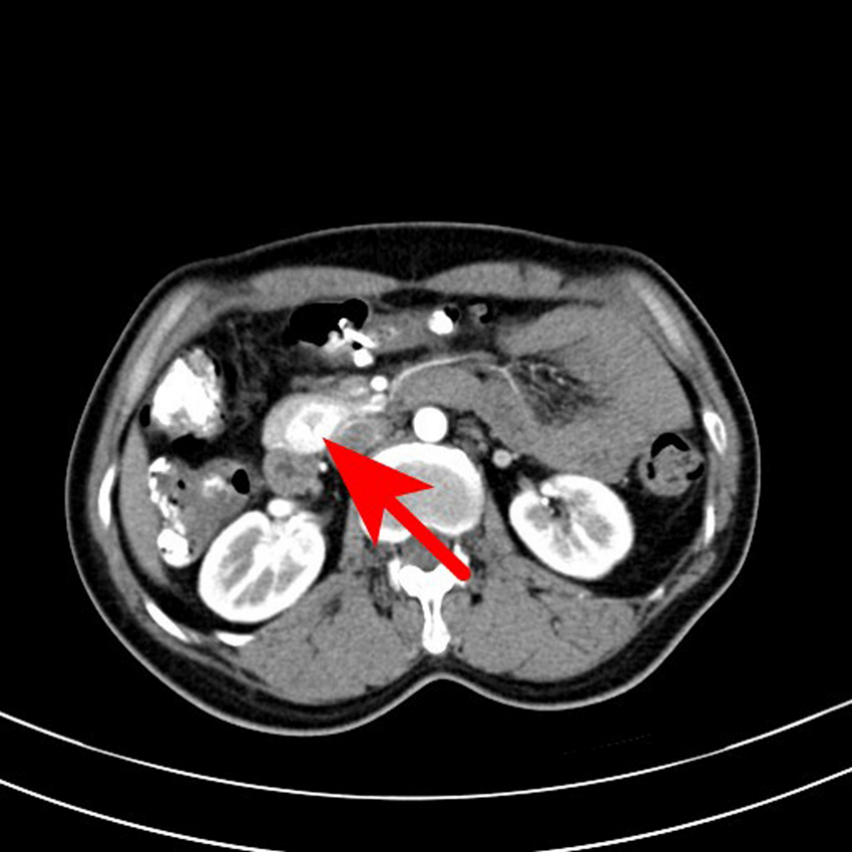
Transverse axial scan of the arterial phase. The lesions showed uneven enhancement, the highest CT value reached 192 HU, and the low-density areas were not enhanced.
